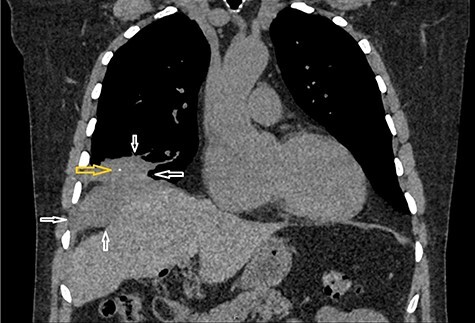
CT scan chest coronal view: Figure demonstrates a mass lesion at the right lung base with an area of calcification. White arrows demonstrate the edges of the mass lesion. Yellow arrow denotes the area of calcification in the mass.
